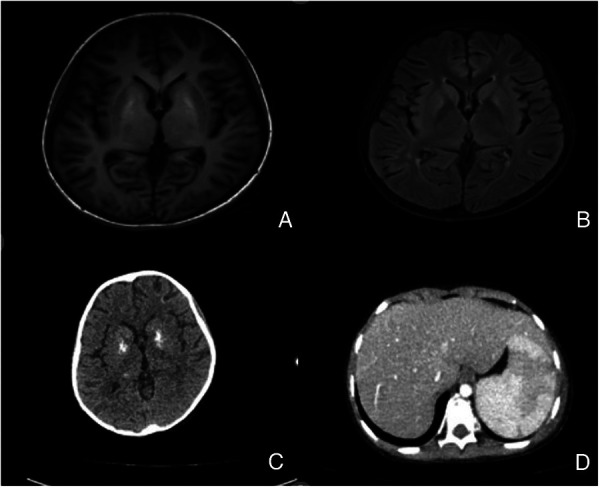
(A,B) T1 and T2 FLAIR imaging suggest the absence of the basal ganglia on both sides, and there are multiple abnormal signals near the ventricles on both sides.); (C) head CT scan shows multiple calcifications in the basal ganglia and ventricles on both sides, suggesting viral infiltration. (D) Abdominal enhanced CT shows extensive infiltration of the spleen.).
